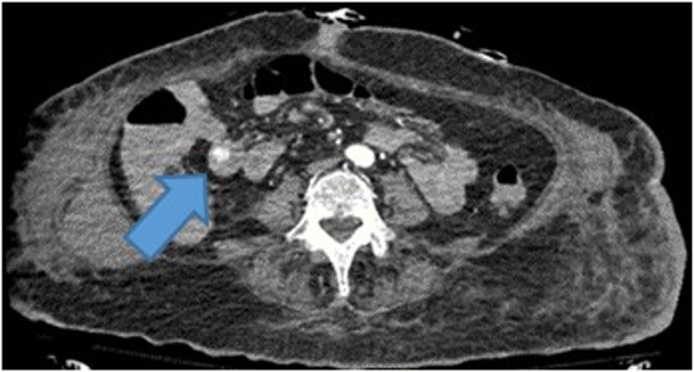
CTA of the abdomen and pelvis demonstrating active bleeding with extravasation of contrast seen within a small bowel loop in the right mid-abdomen, well proximal to the terminal ileum (blue arrow). CTA, computed tomography angiography.
